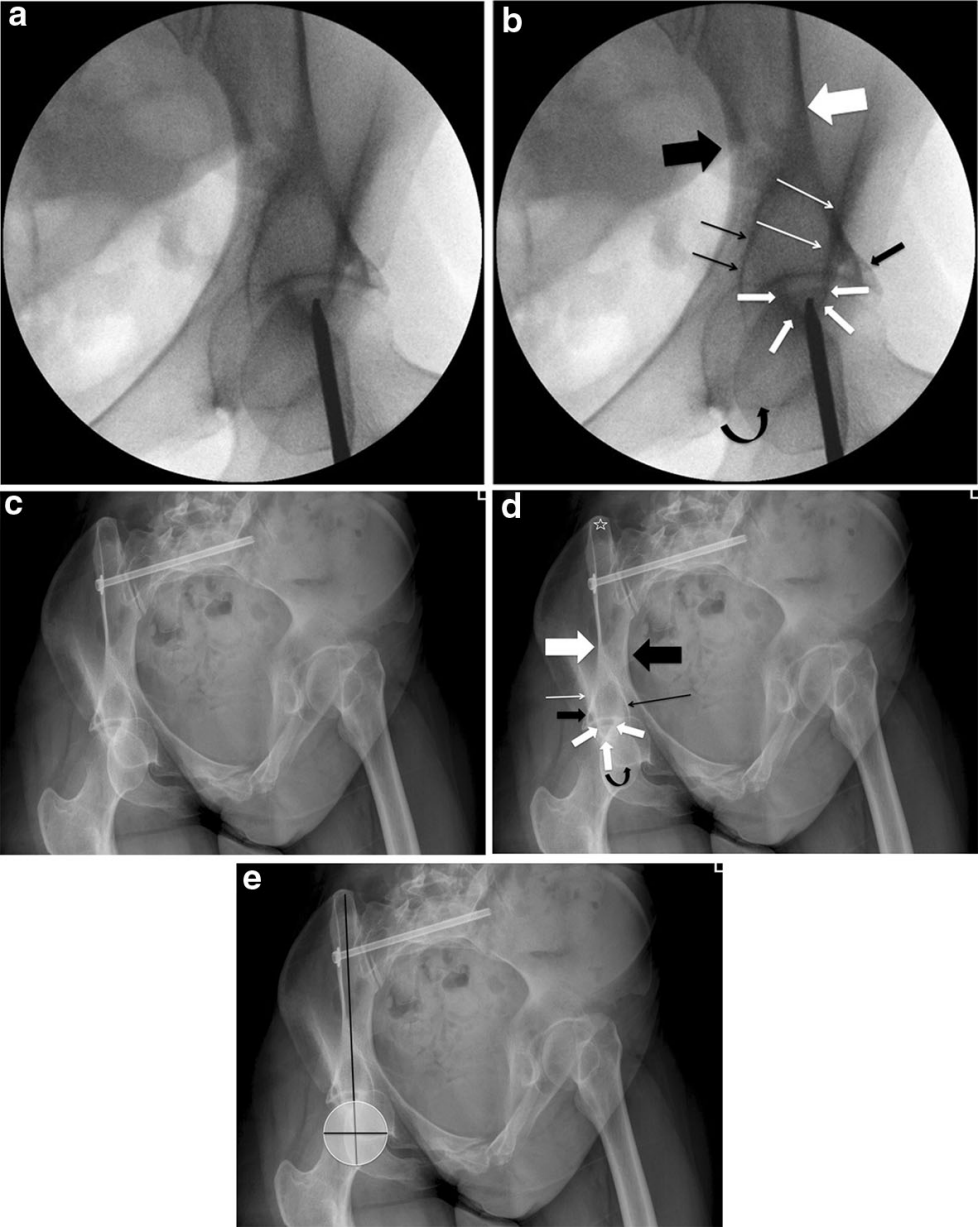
a, b Fluoroscopic and c, d radiographic views of the inlet and obturator oblique views. The following anatomical structures can be identified: anterior inferior iliac spine (intermediate *thickness white arrows*). Inner table of sciatic buttress (*thick black arrow*). Outer table of sciatic buttress (*thick white arrow*). Outer aspect of supra-acetabular area (*thin white arrow*). Inner aspect of the posterior column (*black thin arrow*). Ischium (curved *black arrow*). Posterior inferior iliac spine (*white star*). e In the “ideal IOO view,” the straight line connecting the AIIS to PIIS should bisect the femoral head. The AIIS is projected overlapping with the proximal middle part of the femoral head
